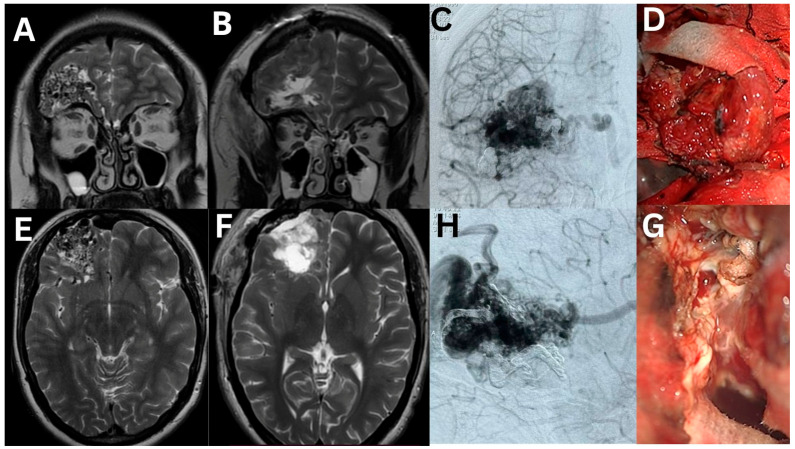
MRI, DSA, and intraoperative findings related to the arteriovenous malformation in the basal sections of the right frontal lobe. The panels include T2-weighted MRI scans (A,E), selective cerebral angiographs before and after pre-embolization (C,H), and macroscopic images intraoperatively (G) and after surgical excision (D). The postoperative T2-weighted MRI studies (B,F) demonstrate the completeness of AVM resection.
